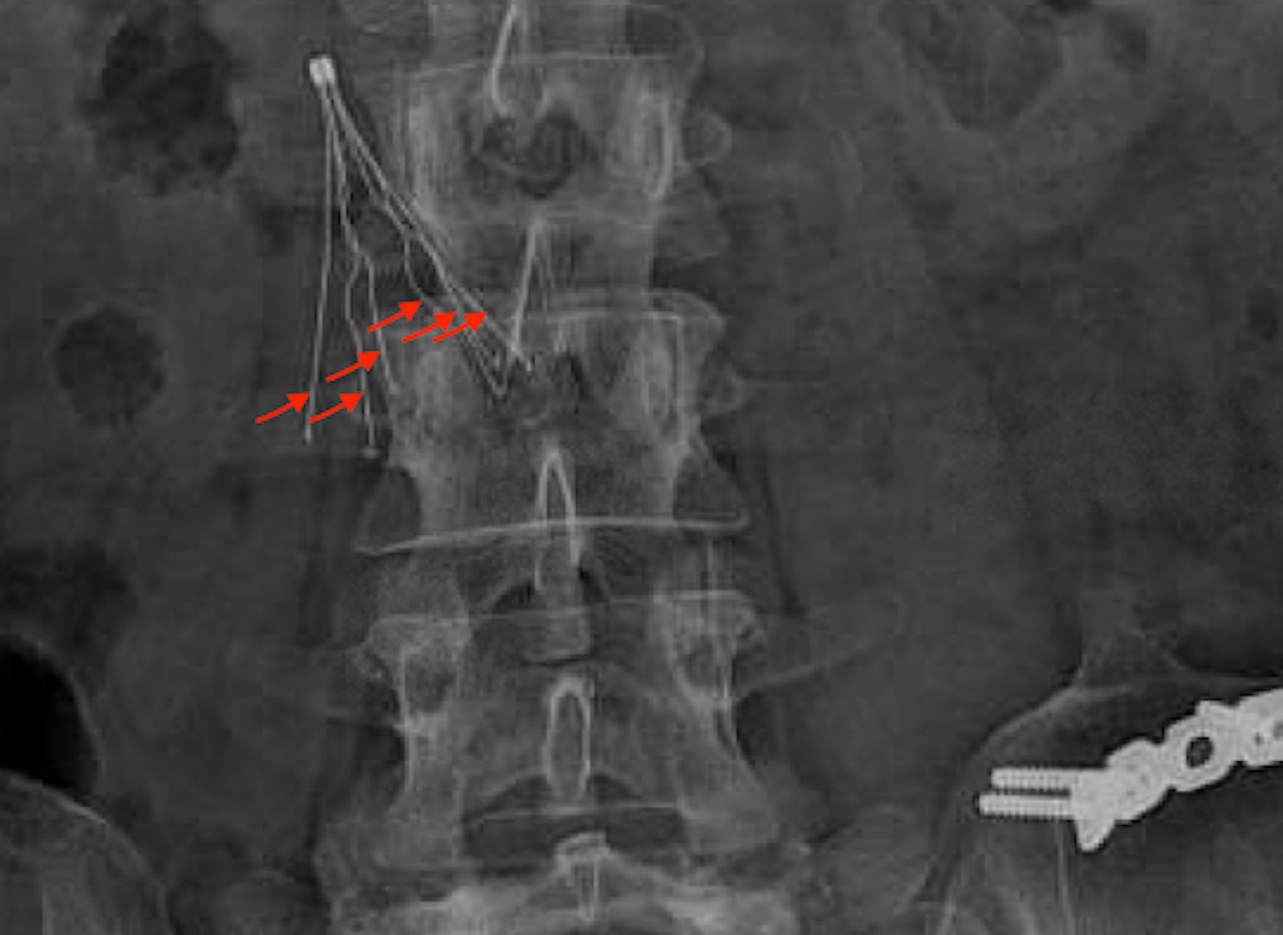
X-ray of the thoracic and lumbar spine showing the inferior vena cava filter with all six struts intact.
